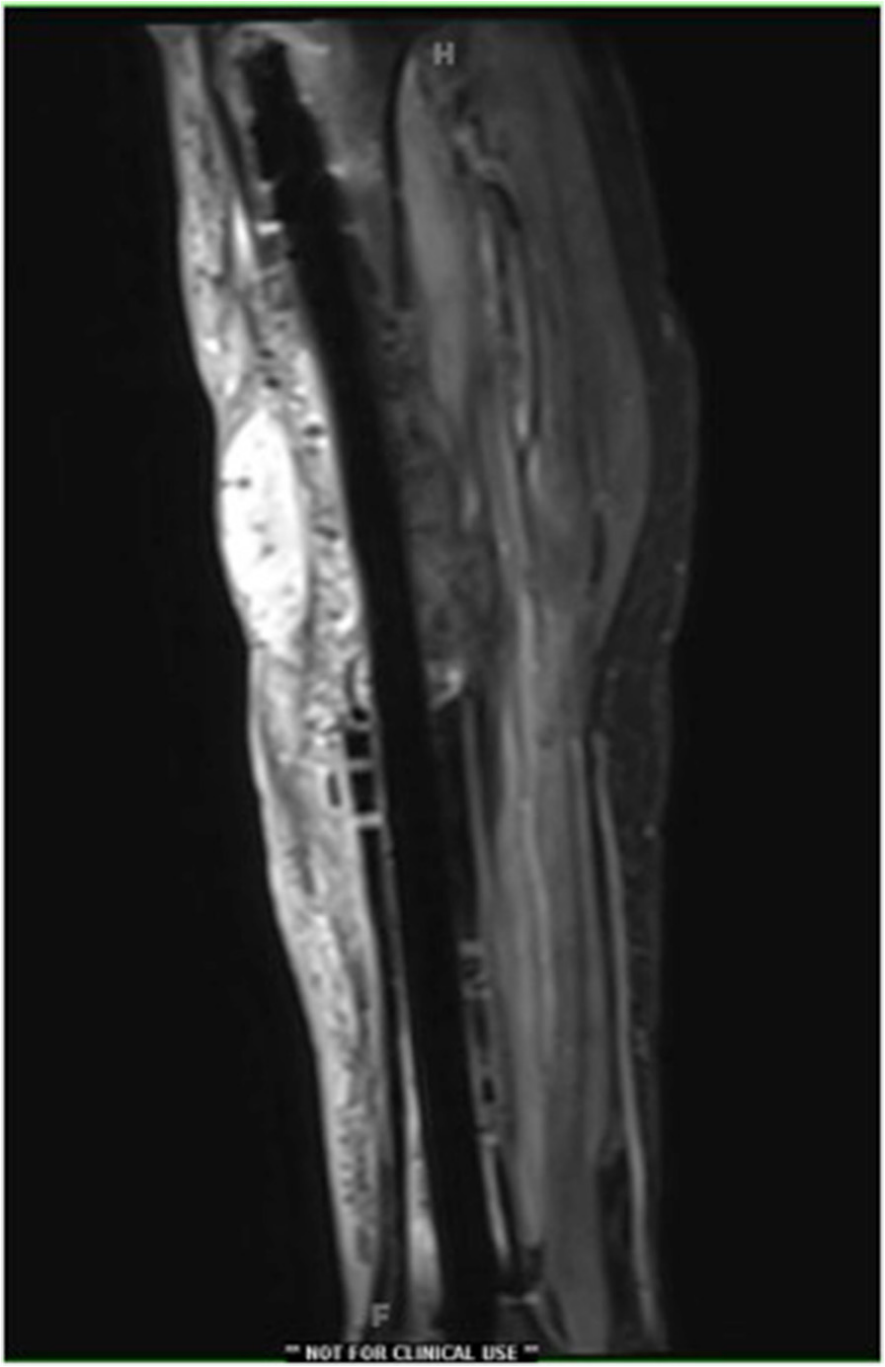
Postoperative MRI of the tibia following definitive fixation with radiolucent antibiotic-impregnated carbon-fiber IM nailing. Use of the carbon-fiber IM nail enables artifact-free MRI visualization
